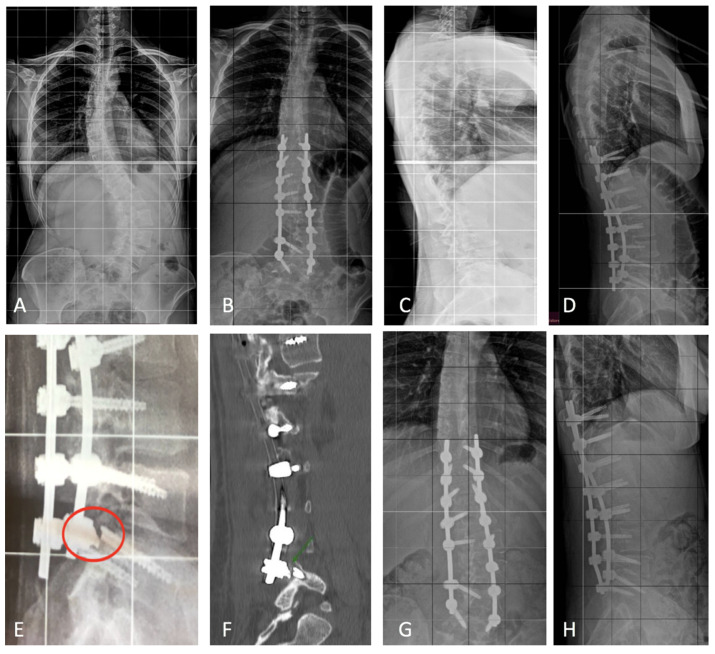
Coronal (A) and sagittal (C) X-ray of a 19-year-old female with Lenke type 5C, who underwent corrective spinal fusion surgery using fenestrated polyaxial pedicle screws (FPSs). The initial surgery aimed to correct the spinal deformity and achieve fusion from T3 to L5, as shown in her post-operative X-rays (B,D). At the clinical check-up at 18 months, the patient complained of severe back pain, and at the subsequent X-ray and CT check-up, she reported failure of the right L5 screw (E,F). We therefore decided to carry out revision surgery. The failed screw was removed and replaced with CPS (G,H). At the 2-year follow-up, no further complications were reported and the patient was in good clinical health.
